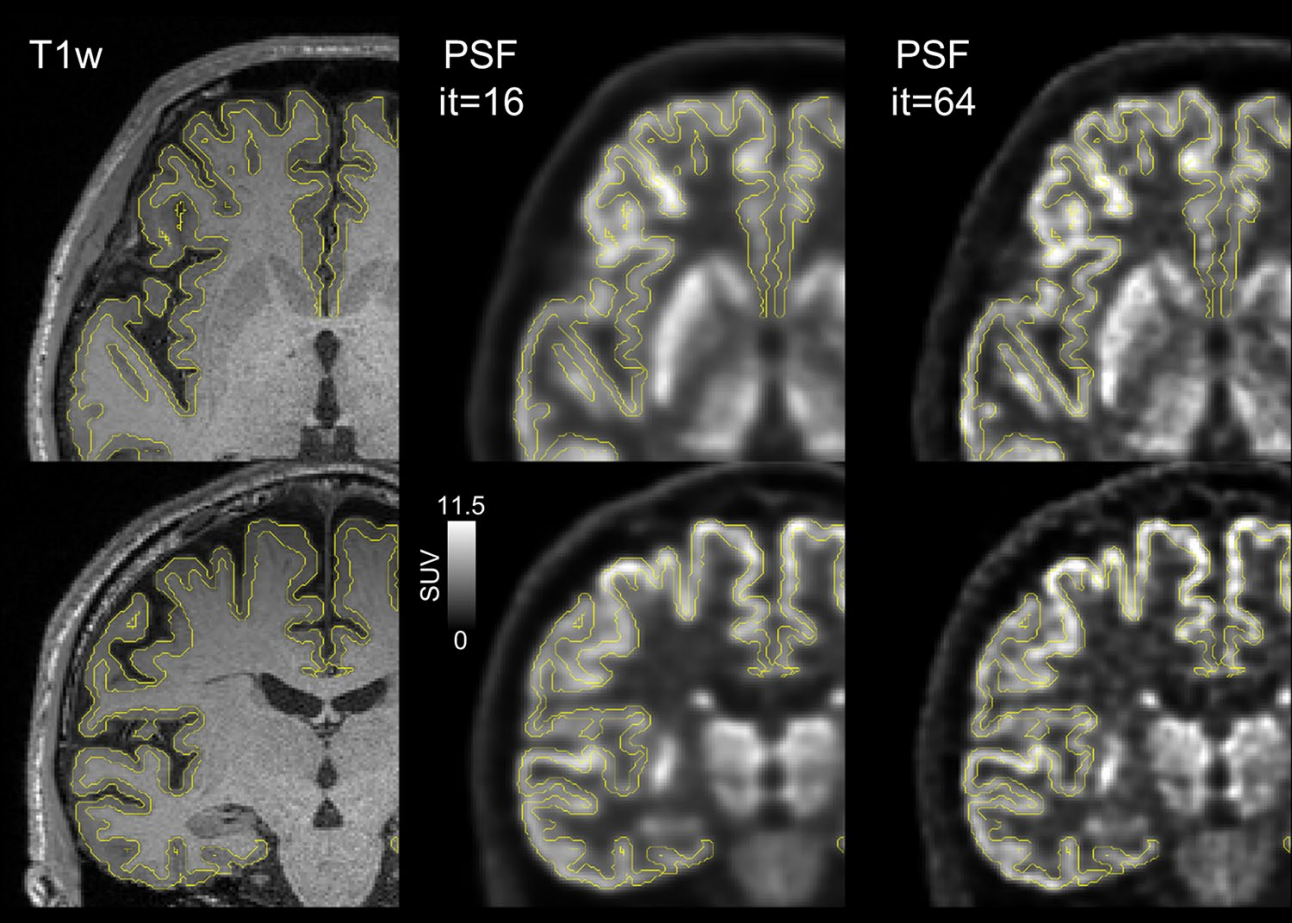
PSF reconstruction images (it = 16 and 64) and MR T1-weighted images from the same subject as in Fig. 2 (subject ID = 1). Overlaid yellow lines represent MR-segmented cerebral cortical boundaries (FreeSurfer)
